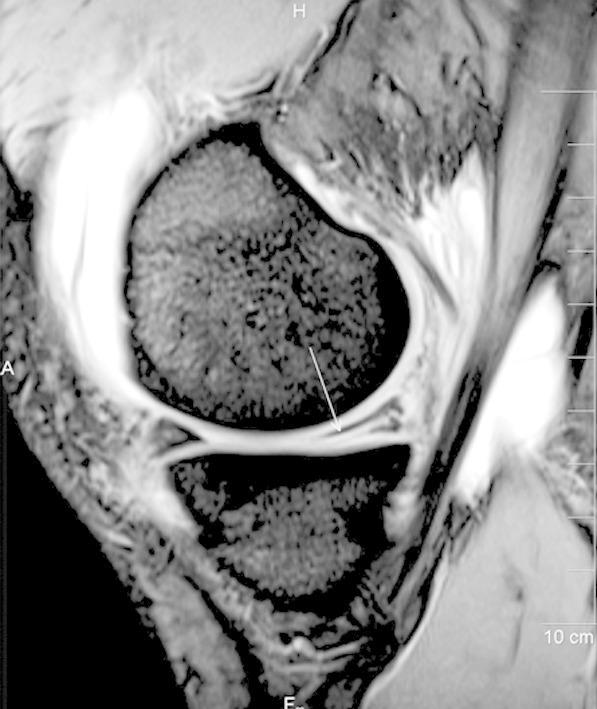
Sagittal T2-weighted MR image revealing grade-3 tear in the posterior horn of medial meniscus. Linear hyperintense signal (arrow) seen extending to the inferior articular surface. Joint effusion also seen in the image.
